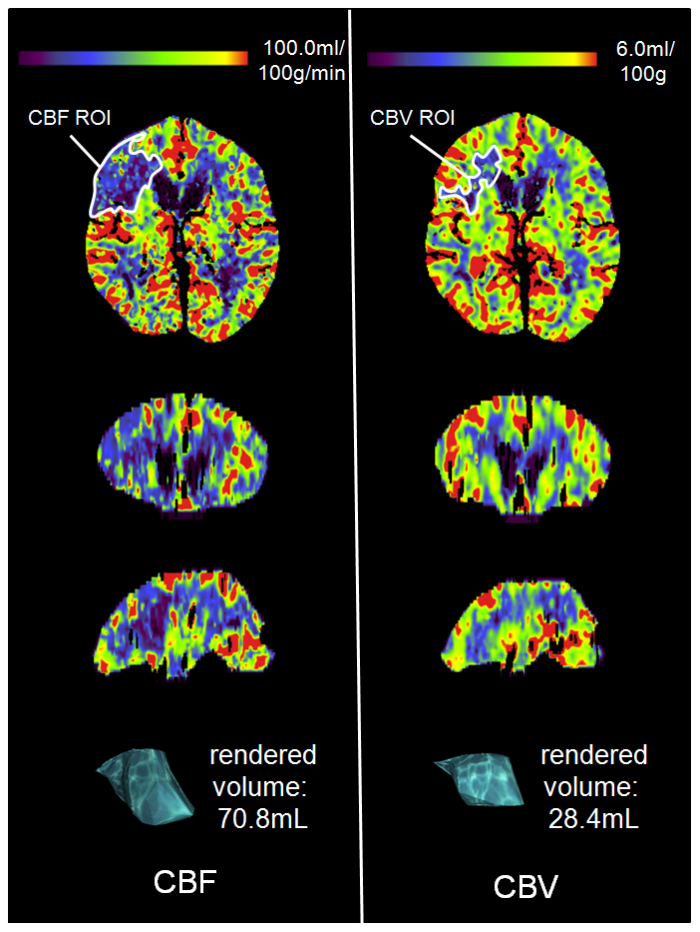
Whole brain CTP mismatch assessment in a 79 yrs old female who presented with a mild left-sided hemiparesis and facial paresis. NIHSS on admission was 2. WB-CTP was performed 185 min after symptom onset. Concerning MM_ASPECTS_, all readers rated for both CBF and CBV ASPECTS regions M1 and M4 in the right hemisphere as the only affected ones. Therefore, in none of the four readings, an ASPECTS mismatch was considered present (MM_ASPECTS_ = CBF_ASPECTS_ - CBV_ASPECTS_ = 8 - 8 = 0). However, volumetric assessment revealed an extensive mismatch of 59.9%. MM_EST_ varied from 30 to 80% (mean 57.5±26.3%). ASPECTS, Alberta Stroke Programme Early CT Score; CBF, cerebral blood flow; CBV, cerebral blood volume; NIHSS, National Institutes of Health Stroke Scale.
